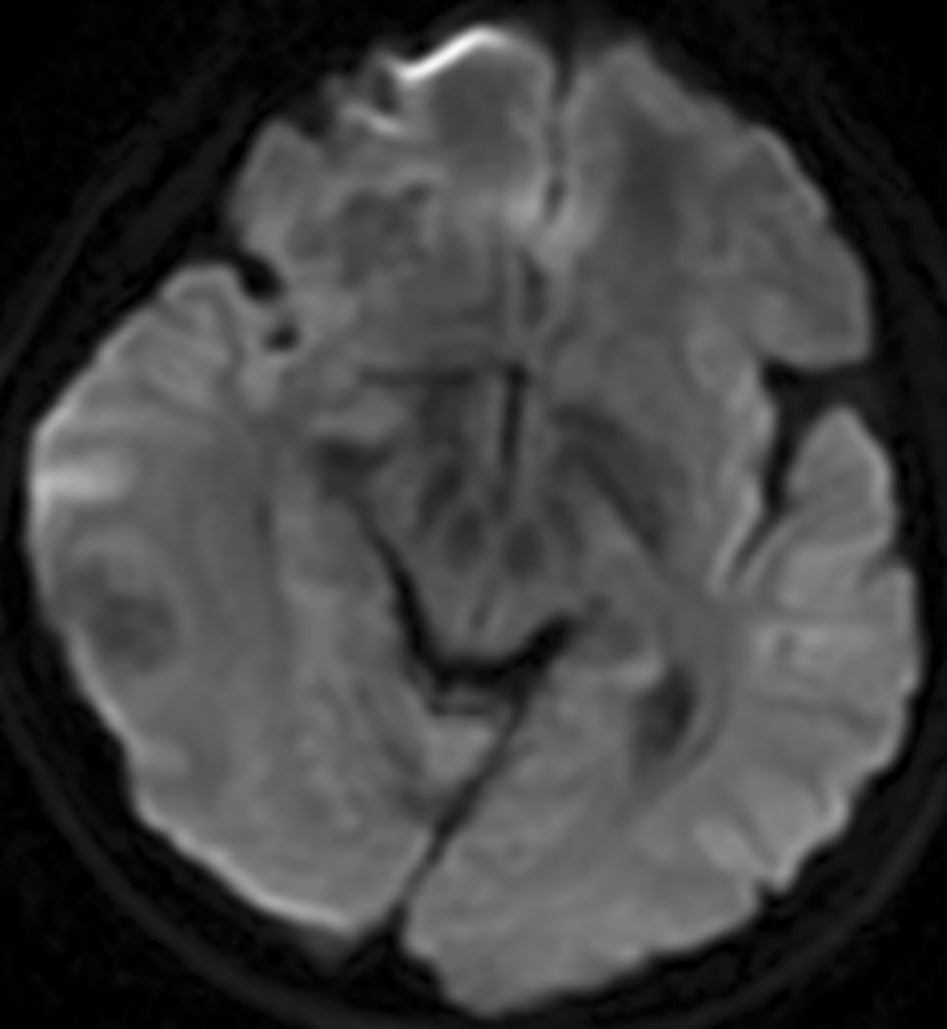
Two lesions were hyperintense signal on a axial MR DWI image.
